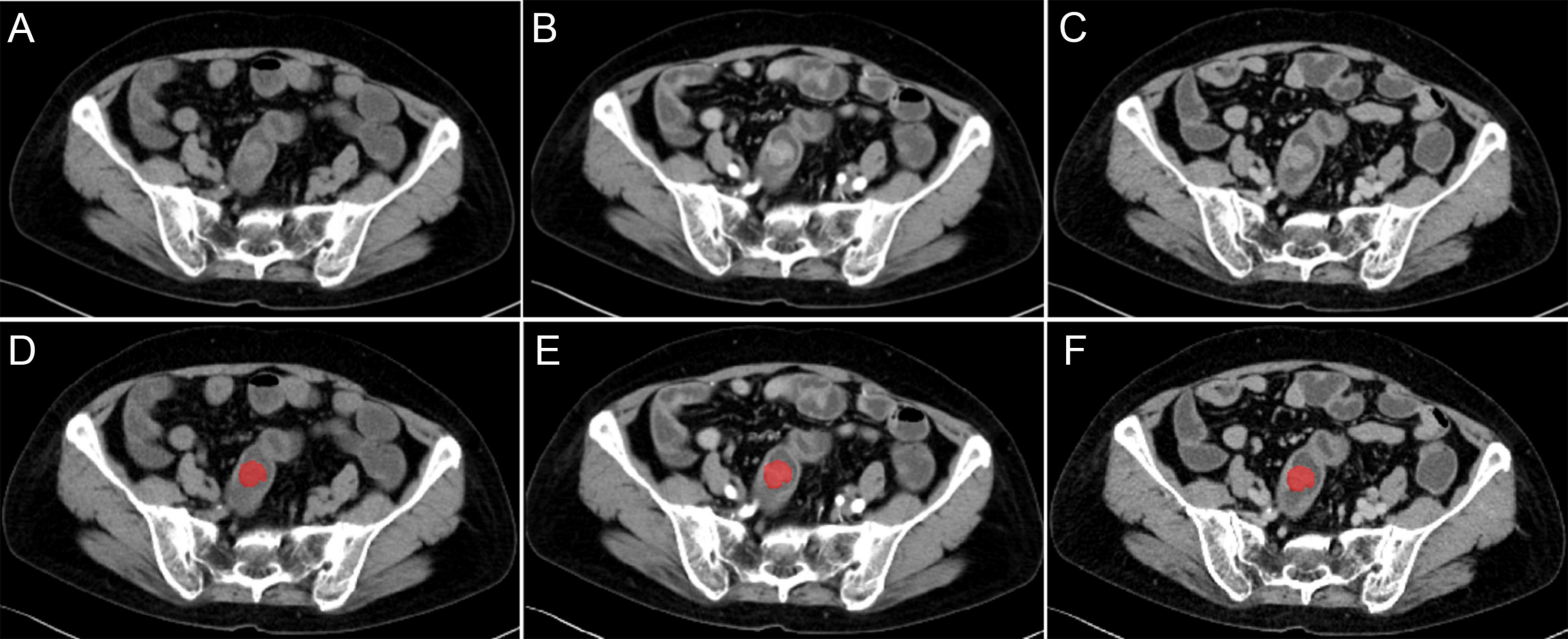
Different CT-phase images used for radiomics analysis. (A–C) Images before the preprocessing of non-contrast phase, arterial-phase, and venous-phase. (D–F) Images after the preprocessing and delineated along the contour of tumor on the largest slices of tumor.
